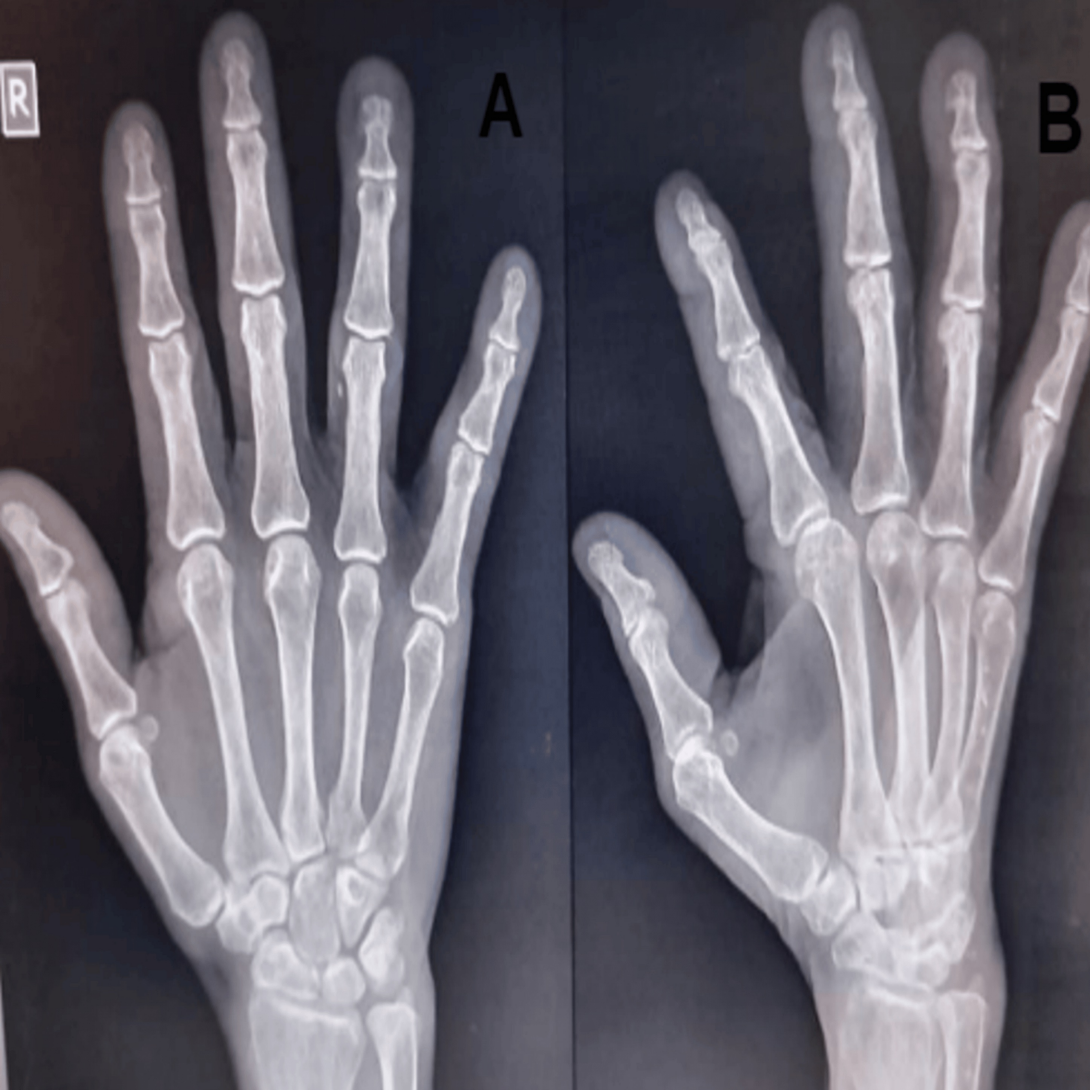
Follow-up X-ray after six months showing no recurrence
